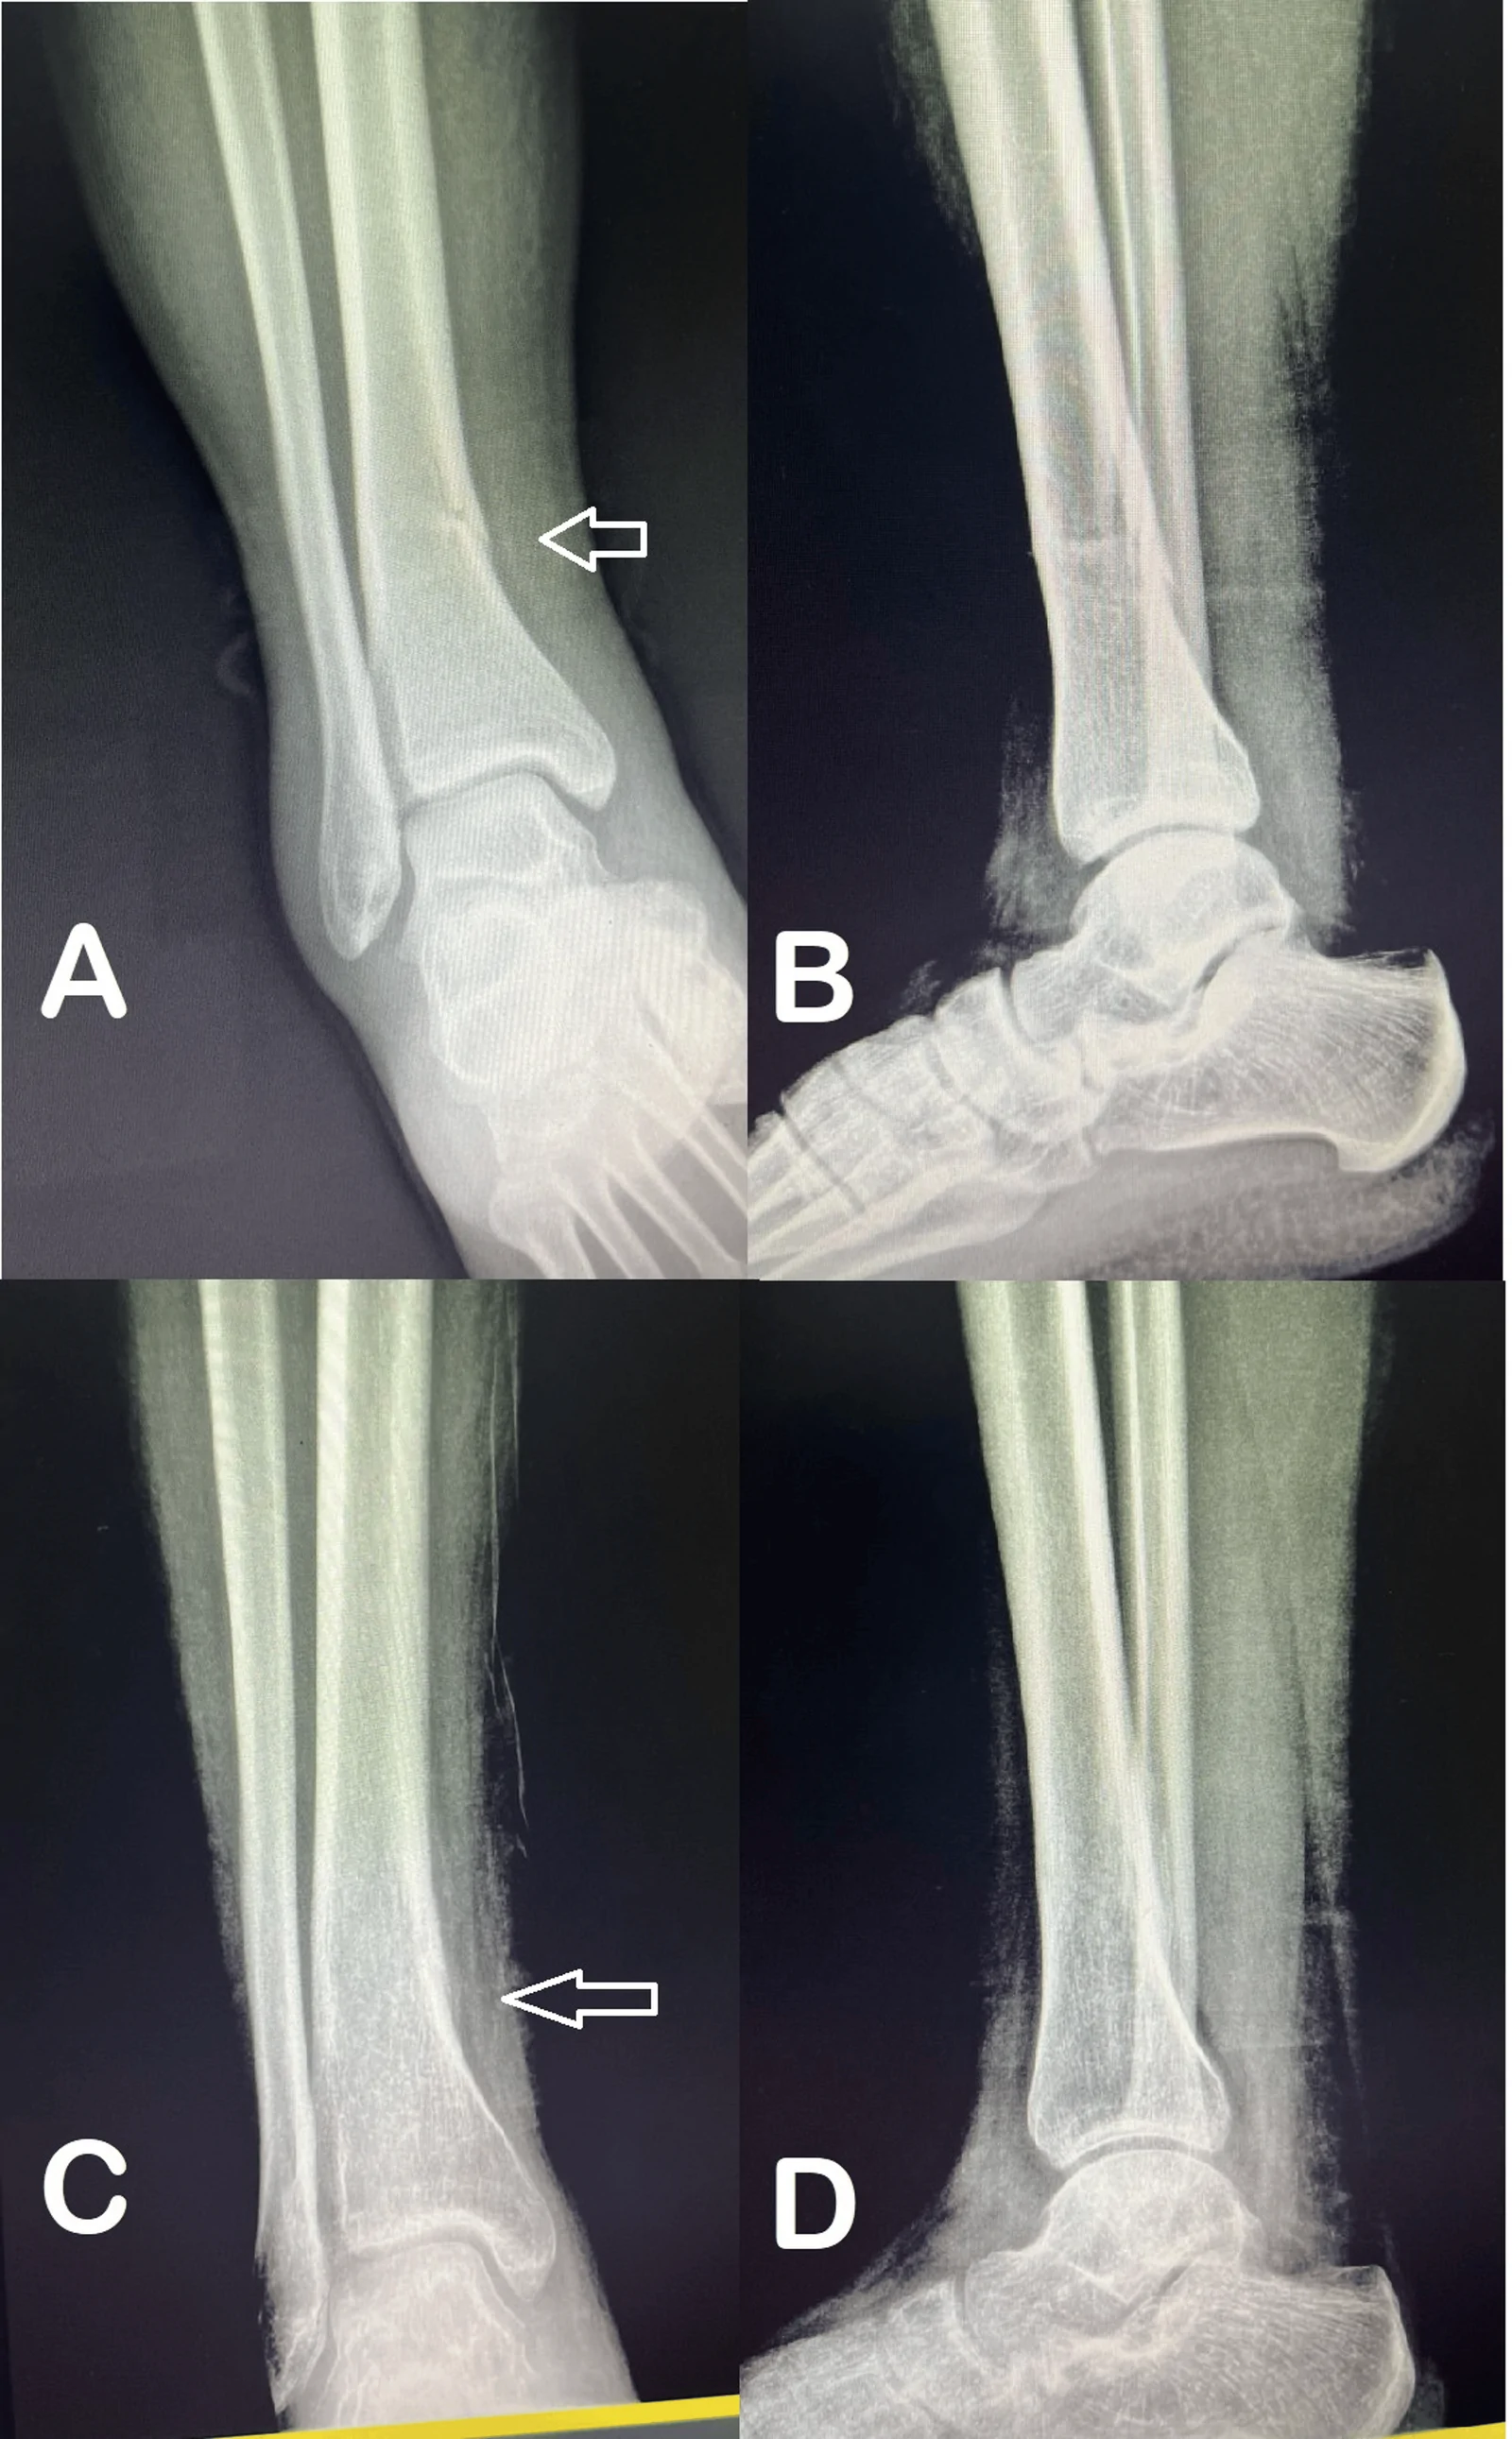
Leg radiographs of a 23-year-old male (A, B) AP and lateral distal leg radiographs obtained at the initial presentation of a 23-year-old male patient complaining of leg pain, showing an incomplete distal tibial stress fracture (arrow). (C, D) Three-month follow-up AP and lateral radiograph views of the same patient showing complete healing of the fracture, which was treated conservatively with a long leg cast, with complete resolution of the symptoms (arrow). AP, anteroposterior.
